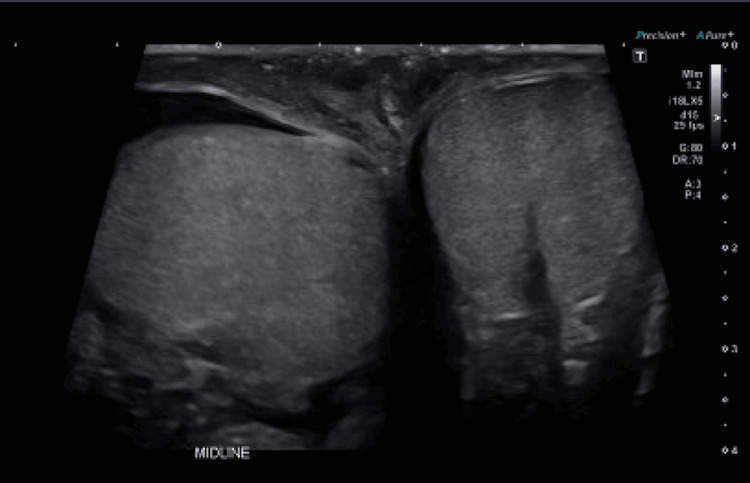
Testicular ultrasound showing no masses
